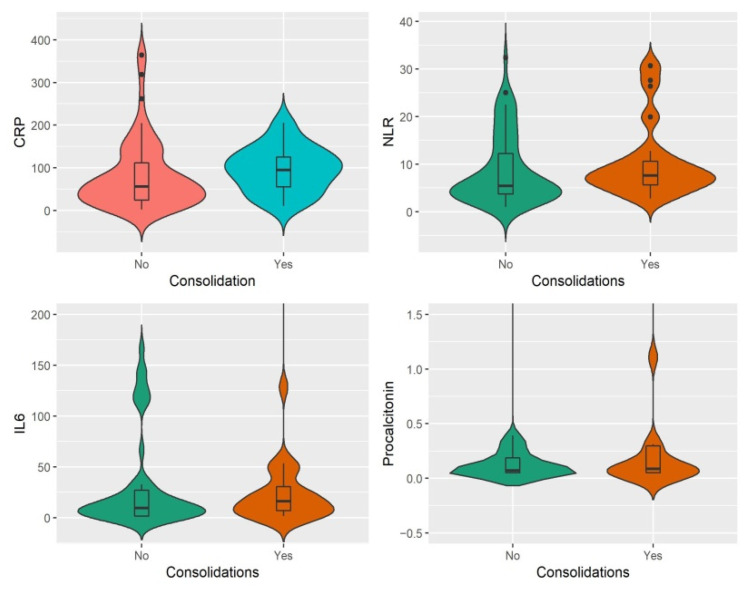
Difference between the medians of inflammatory markers according to CT presence of consolidations.
